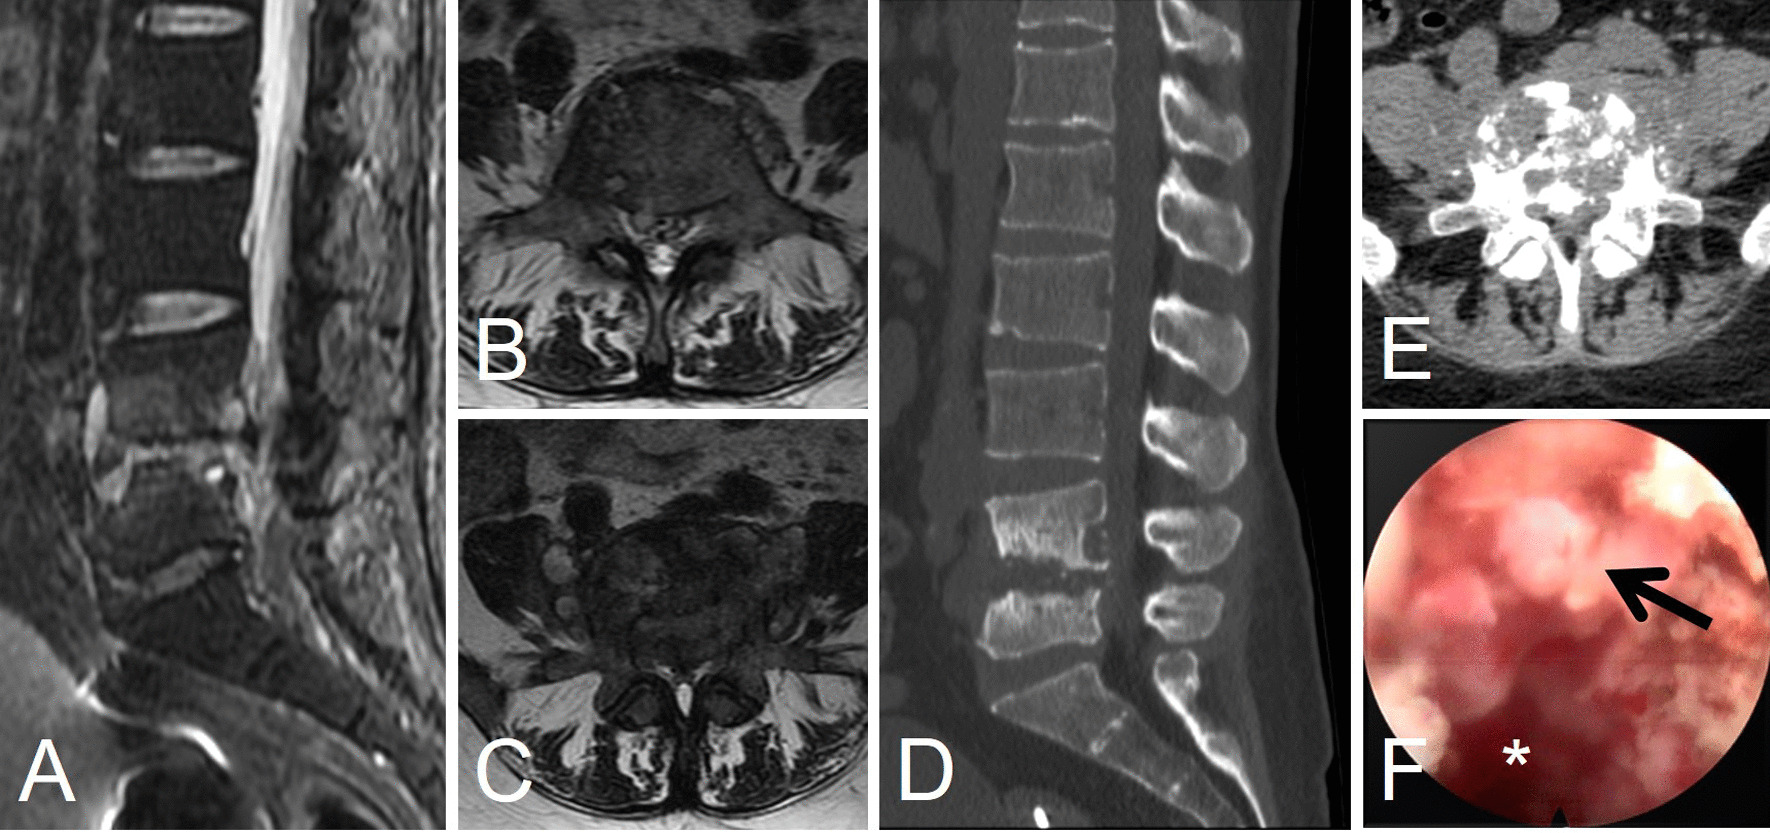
Images of the lesion before or at the first endoscopic surgery (debridement). The MR images of initial administration (A, B), the MR (C) and CT images (D, E) at 5 months after empirical treatment, and endoscopic view of the discal lesion at surgery (F) were presented. The star indicates the interbody space and the arrow indicates inflammatory tissue
